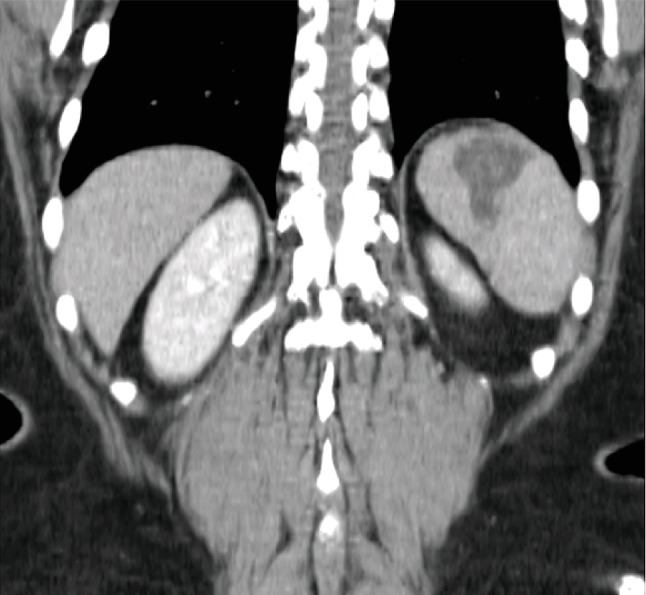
First postoperative CT scan showing an ischemic area of 40 × 40 mm in the superior pole of the spleen.
